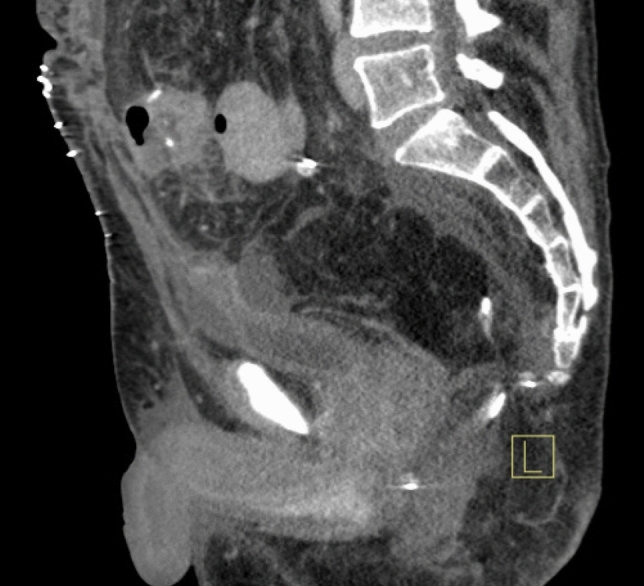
Postoperative computed tomography (CT) appearance of an omental pedicled flap filling the pelvis after pouch excision. Note the partially visible pelvic closed-suction drain, still in situ, positioned in the deepest portion of the pelvis adjacent to the closure of the levators and perineal defect. © Cleveland Clinic Foundation 2025. CT, computed tomography
